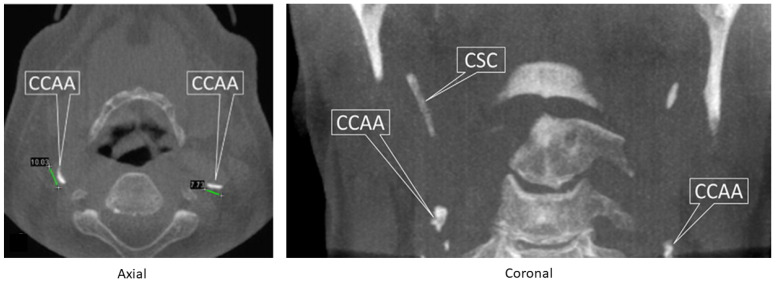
A large field-of-view (FOV) cone-beam computed tomography (CBCT) displaying calcified carotid artery atheromas (CCAA) in the axial and coronal reconstructions (2 different patients). CSC, calcified stylohyoid chain; a normal variant. Adapted from Figures 12.14 and 12.15 in reference [12]; MacDonald, Wiley-Blackwell 2020.
